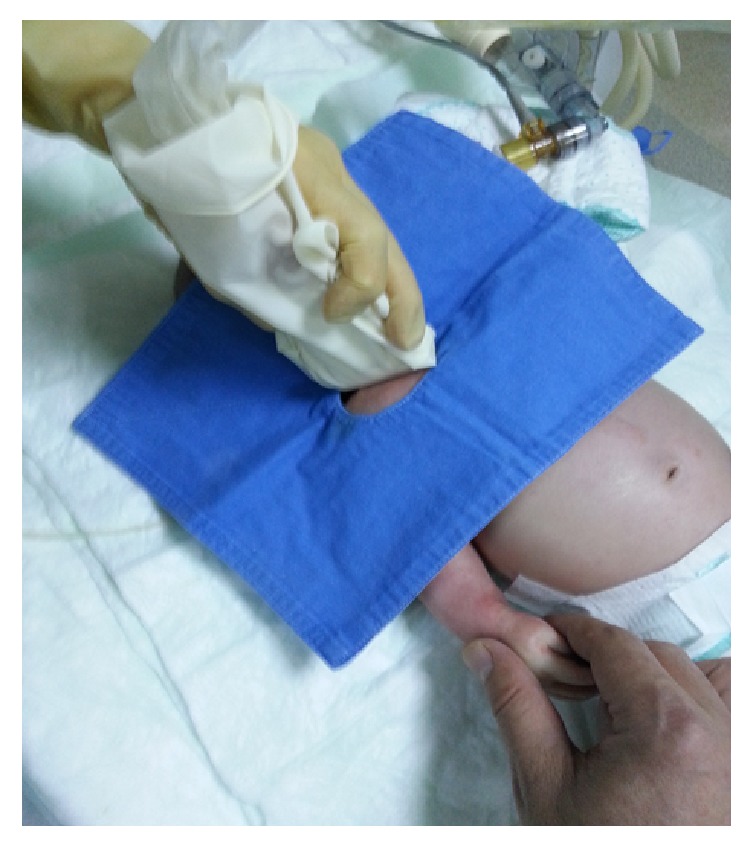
The position of the patient and ultrasound transducer during cannulation.
